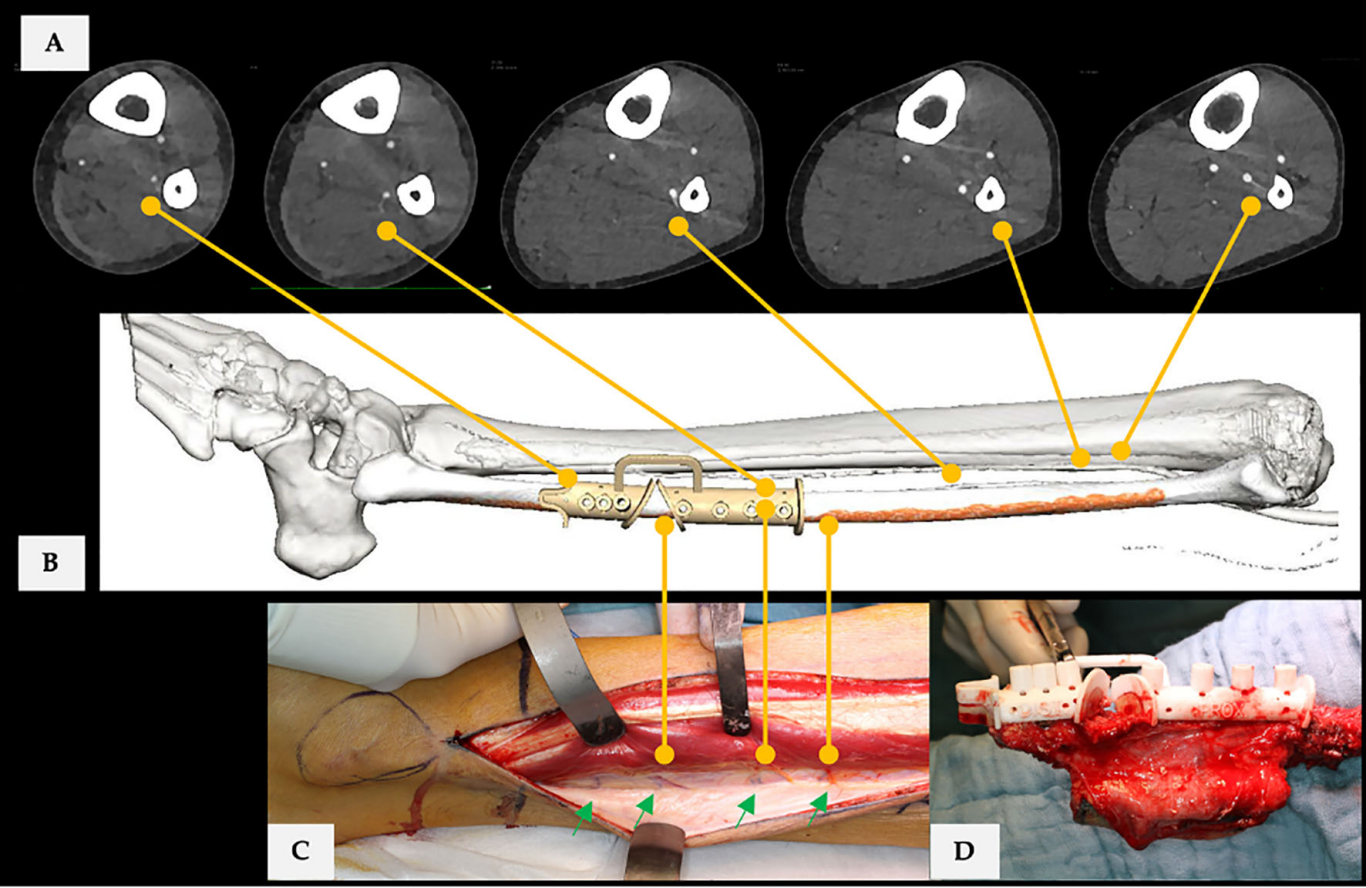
(A) CTA scan (axial plane) of the donor site. Yellow lines connect found PB and SCP with virtual surgical planning. (B) Final virtual surgical planning for bi-segmental mandible reconstruction with free fibula flap. (C) Yellow lines connect corresponding vessels with the operating field. (D) Applied cutting guide, performed osteotomies, and shaped neo-condyle. Case is ID 18 in Figure 5. In CTA assessment were 5 PB and one SCP of the FA at donor site recorded. Each fibula flap segment was supplied by one PB, while three were located proximal to the designed flap. The CTA-based SCP position was in the middle of the skin paddle in the proximal fibula flap segment. The radiological examination of the FA was without pathological findings (type I-B: infra-popliteal branching pattern, which means trifurcation of the popliteal artery in ATA, PTA, and FA). Overall, there were no radiological reservations or restrictions to surgery. Figure 3A, C shows that there was a discrepancy between the radiological and the operative findings. The number of SCP was at least 4 (green arrows).
